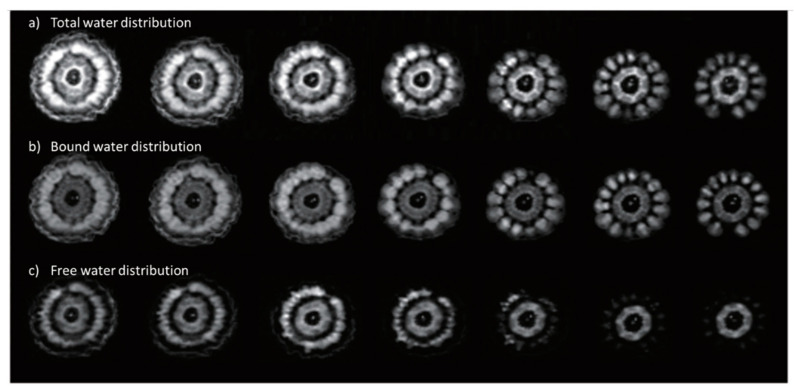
Daily grayscale cross-sectional magnetic resonance images of fresh ear corn (a) total-water, (b) bound-water and (c) free-water, (i.e., expressed as light color intensity) over a week-long drying process. Modified from Wang et al. [25].
